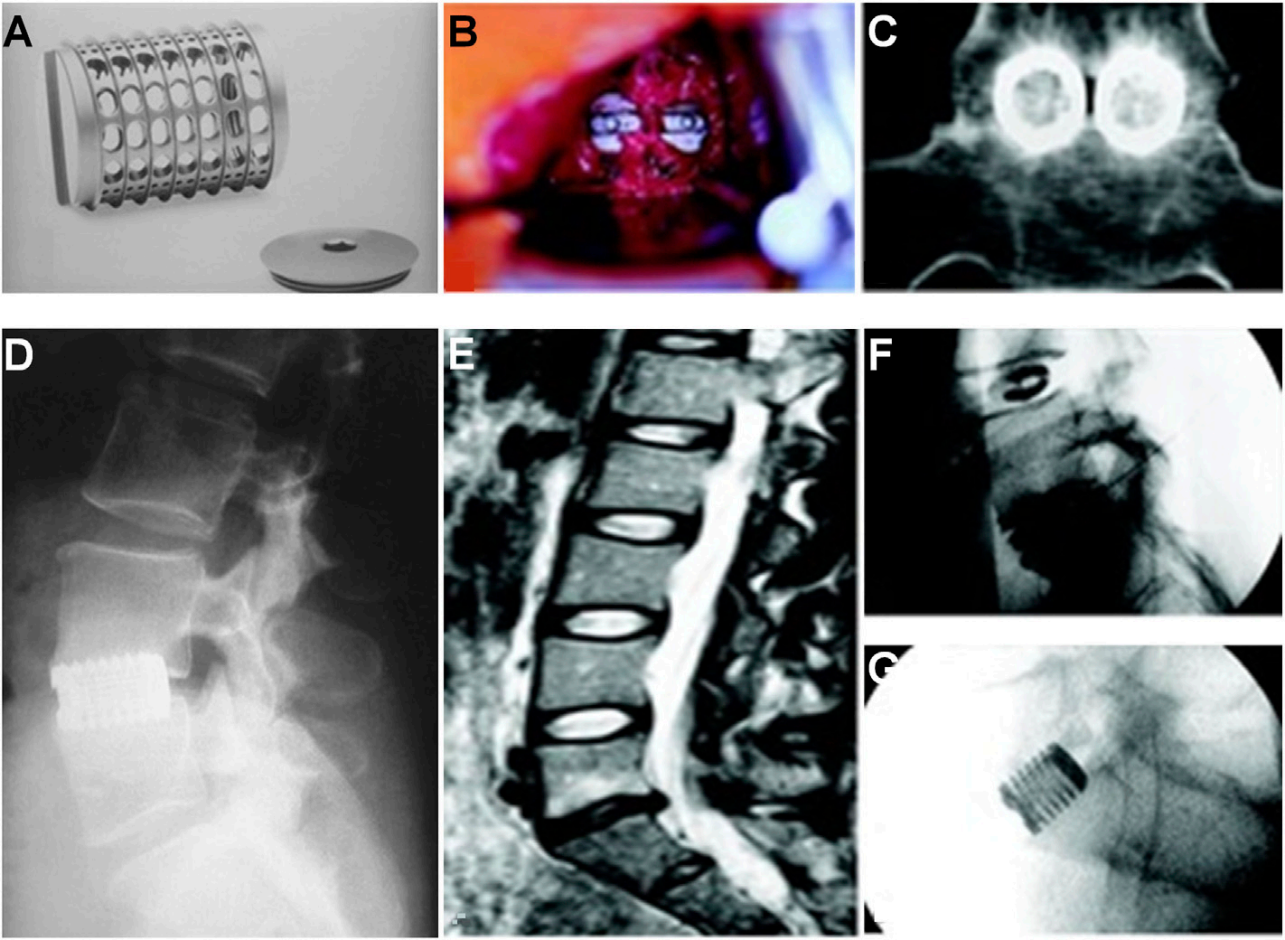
(A) INTER FIX threaded fusion device. (B) Intraoperative picture of the two cages implanted at the L5–S1 interspace. (C) Coronal CT scan 1 year postoperative. Bone has grown through and around the cages. (D) Lateral radiograph of INTER FIX. (E) Preoperative sagittal T2-weighted MRI with degeneration of the L5–S1 disc and a normal L4–L5 disc. (F) Discogram with morphologic abnormality of the L5–S1 disc and a large posterior anular tear. The L4–L5 disc is anatomically normal. Exact concordant 10/10 pain was reproduced at L5–S1. The L4–L5 injection caused no pain. (G) Postoperative lateral radiograph with INTER FIX cages in good position (Sasso et al., 2004).
